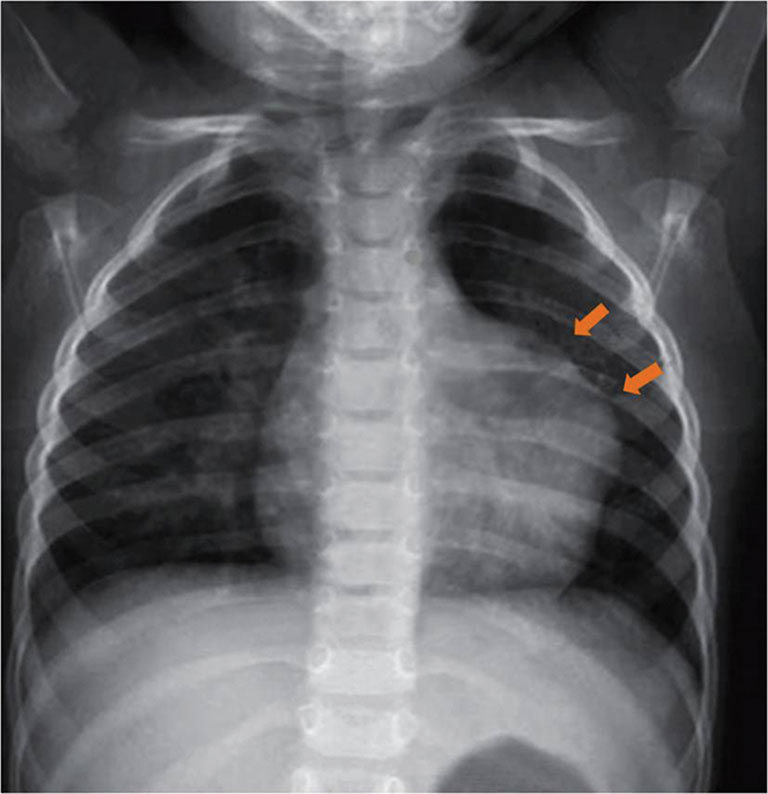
Frontal radiograph of the chest reveals cardiomegaly with a prominent convexity (arrows) of the upper left heart border. The lung fields are unremarkable.
